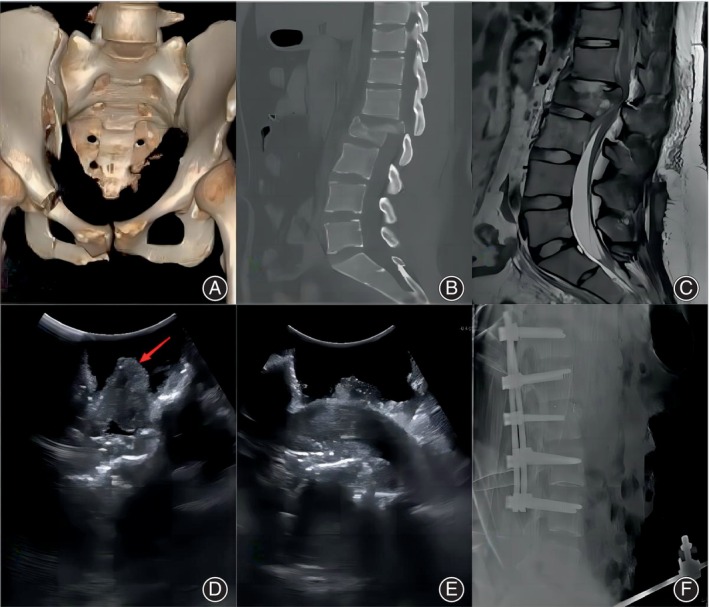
Case 2: Three‐dimensional computed tomography (CT) of pelvis showed unstable pelvic fractures (A). Spine CT and magnetic resonance imaging showed L2 burst fracture, and the fracture fragment encroach spinal canal compressing dural sac and cauda equina (B, C). In the lateral decubitus position, intraoperative ultrasound showed that cauda equina nerve herniated from the dural sac (D red arrow), the echo of conus medullaris and cauda equina was homogeneous. A subsequent ultrasound examination showed complete decompression of the spinal canal (E). Postoperative follow‐up radiographs showed satisfactory reduction of the fracture and a good internal fixation position (F).
